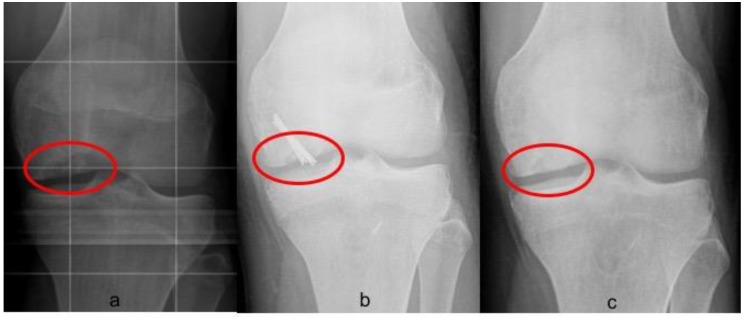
Radiographic union of grade III Osteochondritis dissecans (OCD) of 2.3 cm^2^ in the medial condyle of left knee fixed with two Herbert screws. (a) Preoperative evaluation on anteroposterior (AP) view; (b) two months postoperative X-ray, not complete union was appreciable; (c) six months postoperative X-ray showing a complete union.
